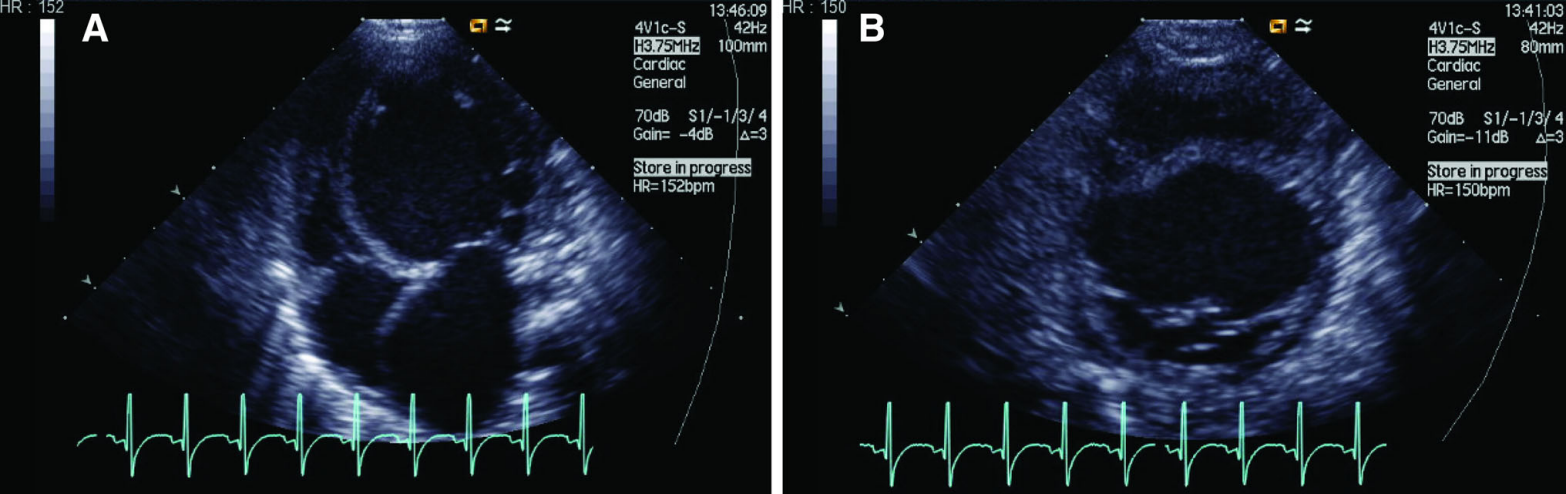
The echocardiogram at the time of presentation in the proband. a Apical four-chamber view showing the enlarged left ventricle. b Short-axis view showing the enlarged left chamber
